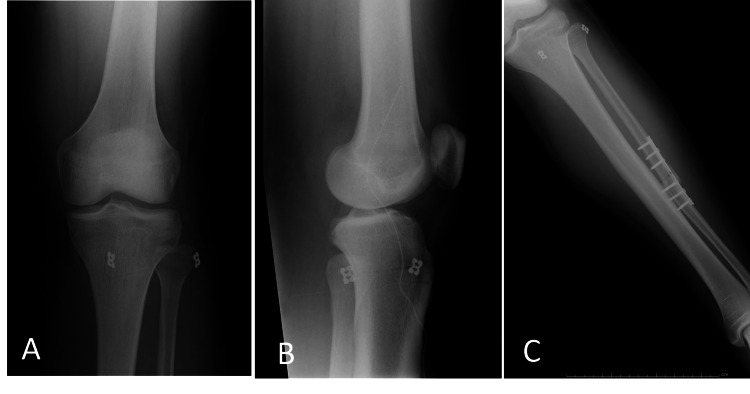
Postoperative X-ray radiographs (A, B, C) Postoperative X-ray radiographs (anteroposterior (A) and lateral view (B) and anteroposterior view of the leg (C)). Dislocation of the proximal tibiofibular joint is reduced.
